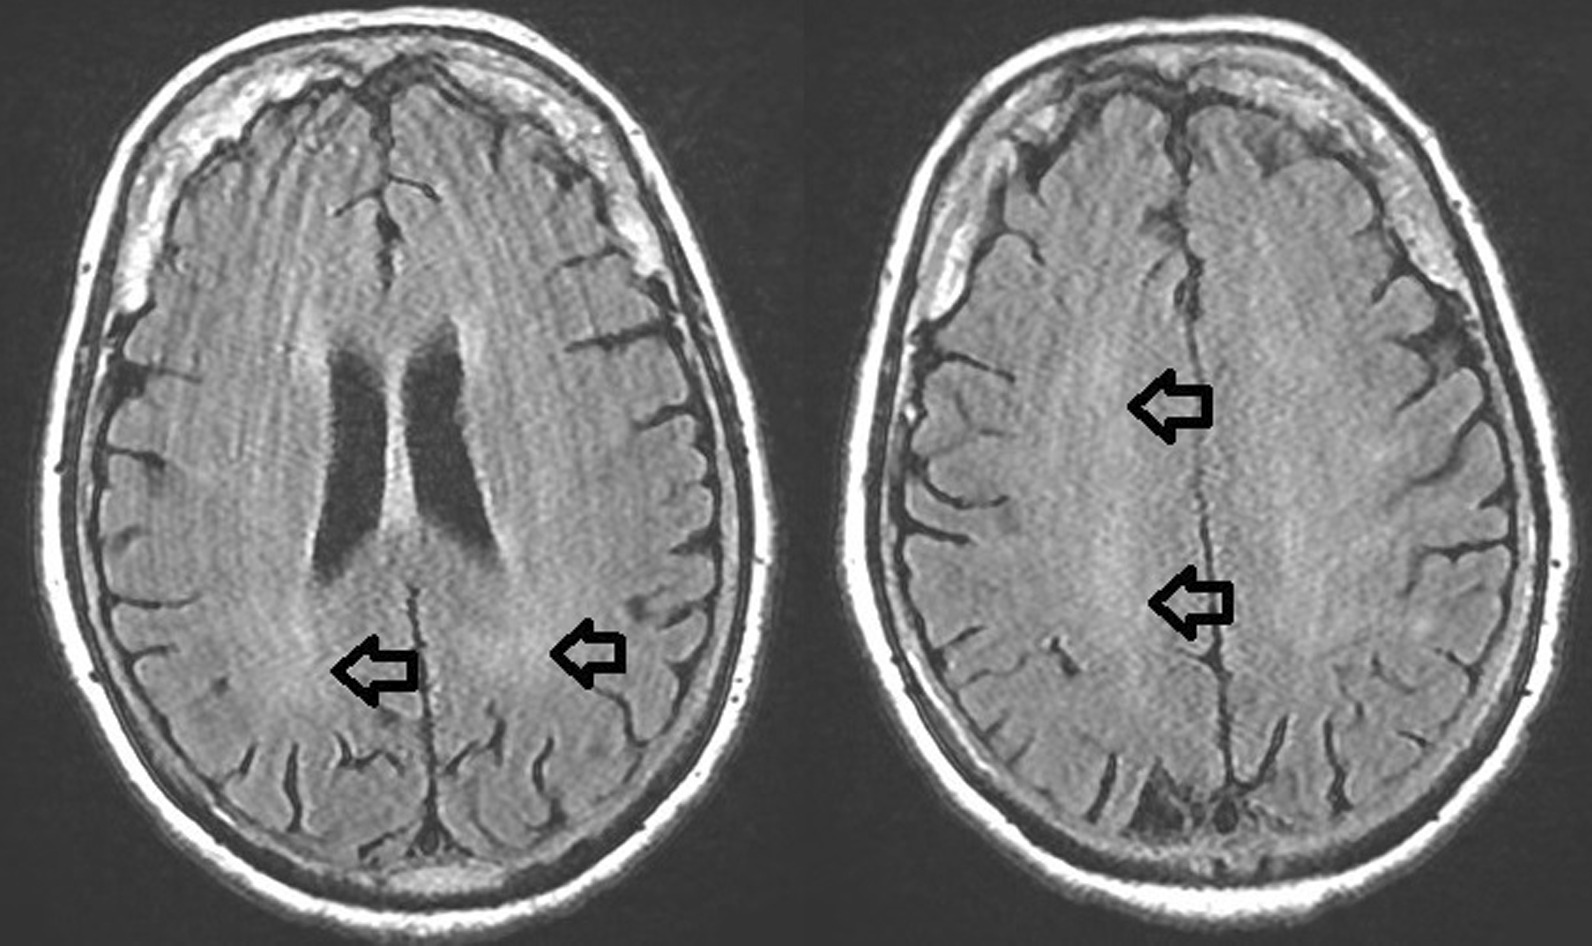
Patient#3’s brain MRI performed at 16 days from symptoms onset, showing lobar white matter hyperintensities (black arrows) on T2-FLAIR sequences. Motion artifacts are present
